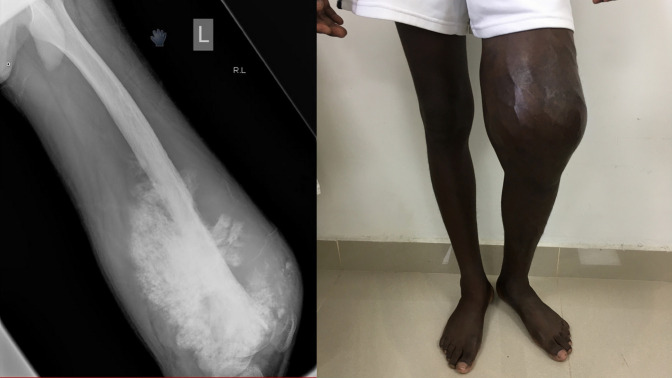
Lateral radiograph of the left femur showing a lesion of the distal femur with bone formation and a sun burst pattern characteristic of osteosarcoma. Clinical photograph demonstrating associated swelling and deformity about the distal femur. Limb salvage was not chosen due to the risk of the surgery, lack of chemotherapy, and lung nodules on chest radiographs.
